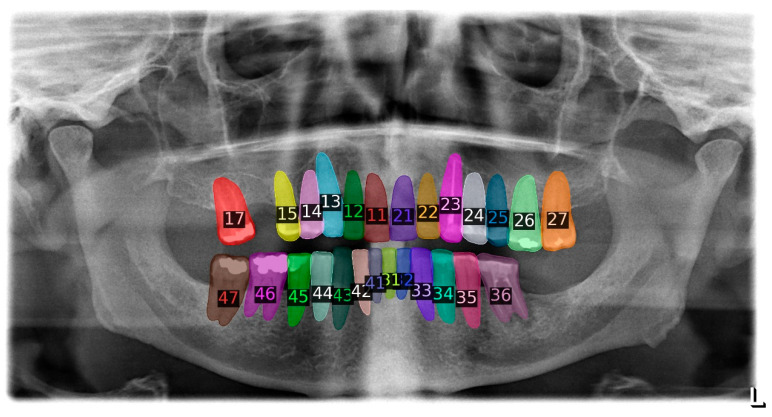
Panoramic radiograph analyzed by the AI-based tooth detection system.
